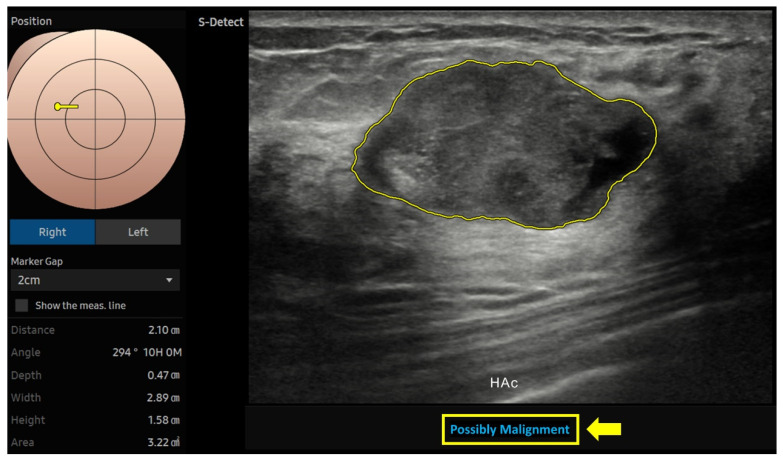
An AI-generated region of interest (ROI) was delineated around the mass’s border. S-Detect automatically extracted and displayed ultrasound features based on the ultrasound BI-RADS lexicon. The final determination based on the high-accuracy (HAc) mode was categorized as either “possibly benign” or “possibly malignant” (arrow).
